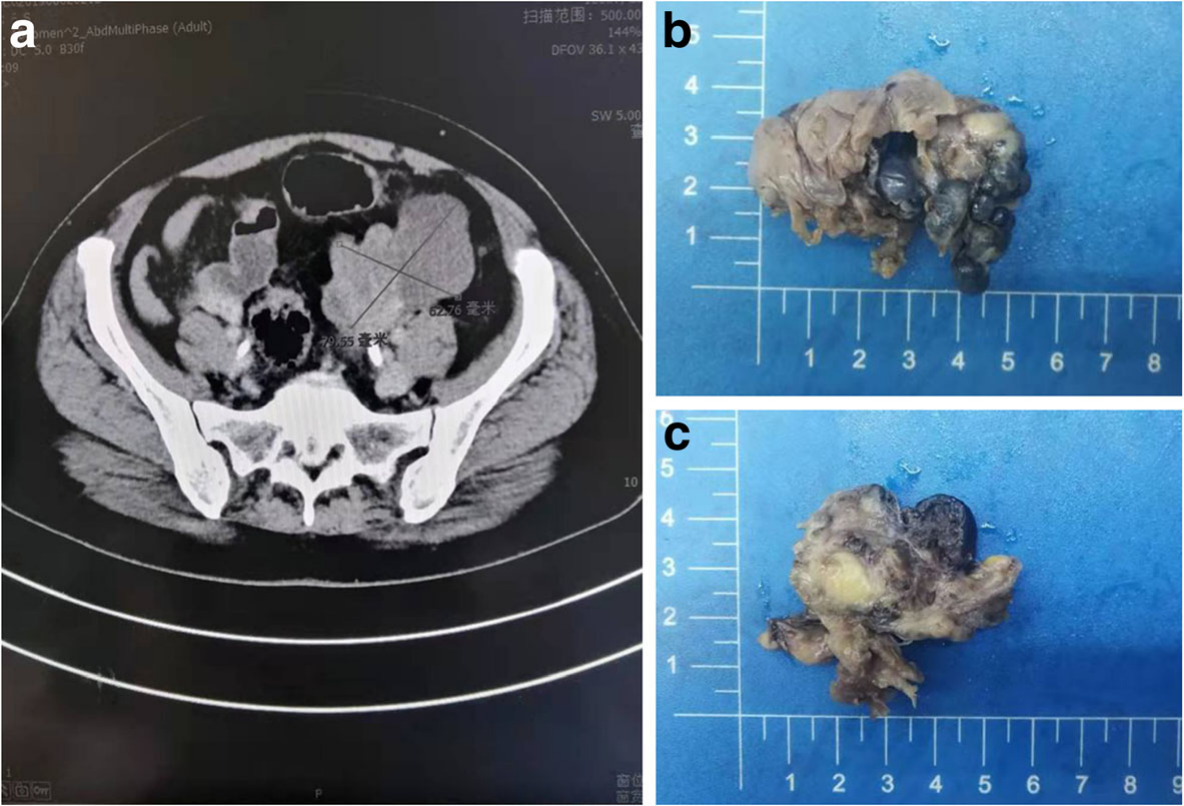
CT findings and macroscopic observation for the tumor mass. a; CT scan revealed an irregular soft tissue density mass shadow in the left appendix area, with a size of 7.9 × 6.2 cm. The mass was nodular and lobulated with clear edges. The unevenness of the lesion was significantly enhanced after enhanced scanning. b: Grossly, the left ovary was enlarged and sized 5.5 × 4.5 × 4 cm with nodular appearance and gray-white to atropurpureus surface. c: Some areas of the cut surface were solid and gray-white with intermediate texture, and some areas were spongy and atropurpureus with soft texture
